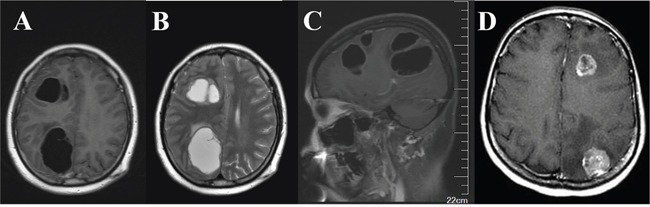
Typical characteristics of cystic versus solid brain metastases observed in MRI images Axial T1-weighted (A) and T2-weighted (B) post-contrast MRI of right frontal and parietal cystic metastases with appearance of hypointensity A. and hyperintensity B. and surrounding edema. C. Sagittal T1-weighted post-contrast imaging. D. Axial T1-weighted MRI of solid lesions.
